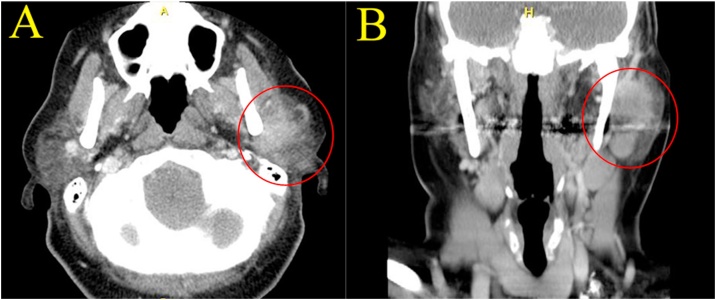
Computed tomography images: A. Axial slice showing a heterogeneously enhancing left parotid mass with deep lobe involvement B. Coronal reconstruction showing the same enhancing mass.
